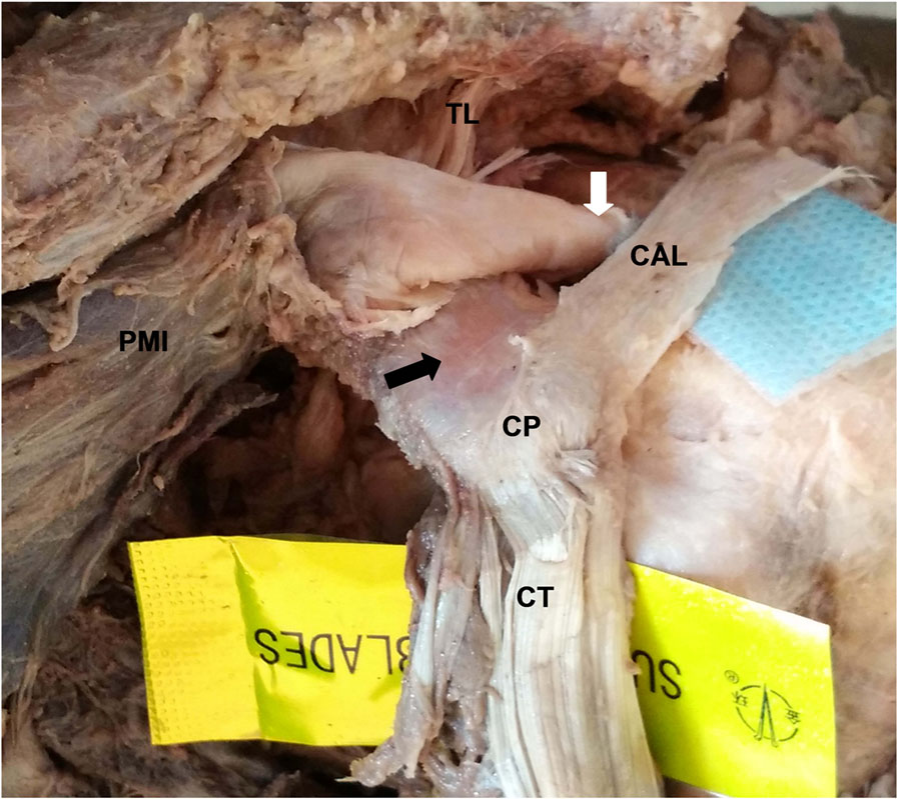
Anatomical structures associated with the isolated coracoid process of the left shoulder with emphasis on the variation. *Black arrow* indicates the coracoid acting as a lever for the pectoralis minor as the muscle passes over it. *White arrow* indicates the ligament of the pectoralis minor, deep to the joint capsule of the glenohumeral joint. *CAL* coracoacromial ligament, *CP* coracoid process, *CT* conjoint tendon, *PMI* pectoralis minor, *TL* trapezoid ligament
